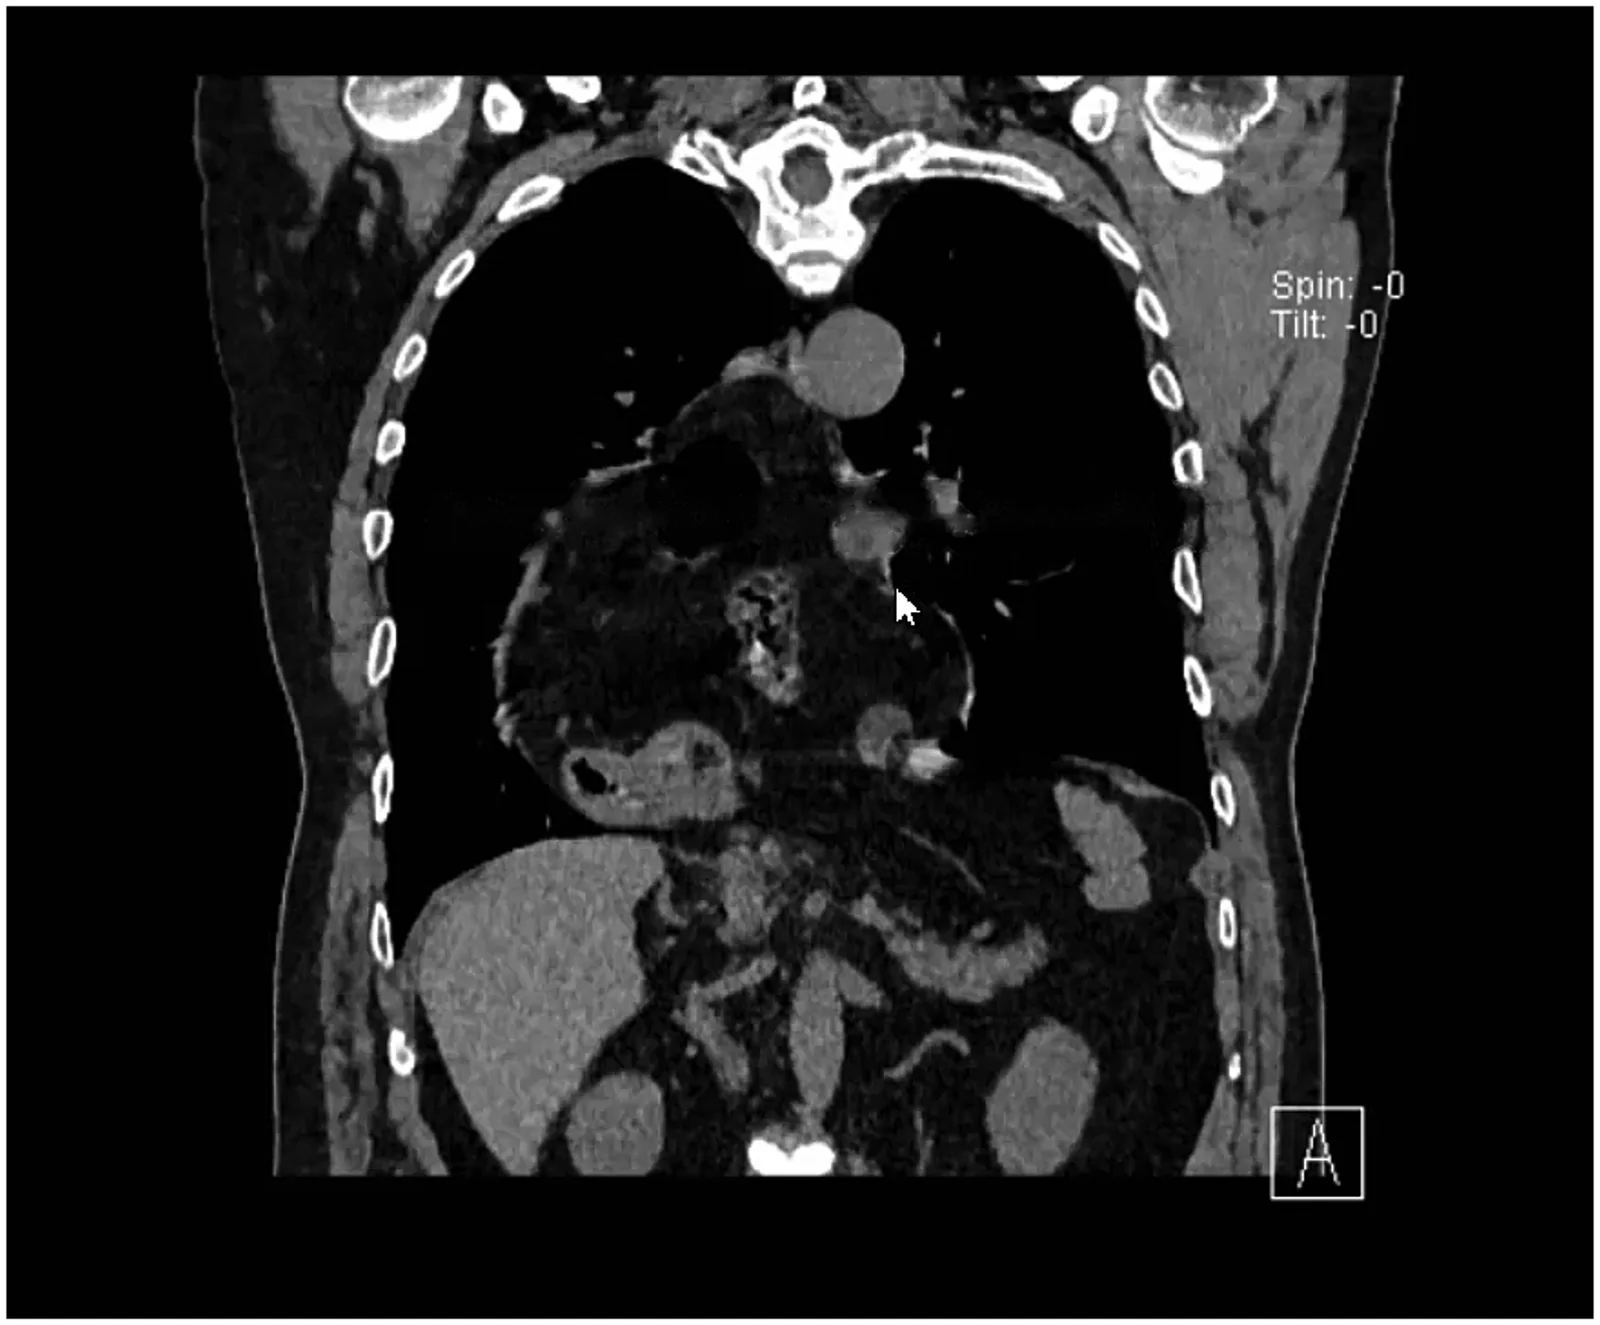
Multi-slice CT (coronal). Giant Type IV paraesophageal hernia (20 × 23 cm hernia sac) with stomach, colon, and small bowel within the thorax.
